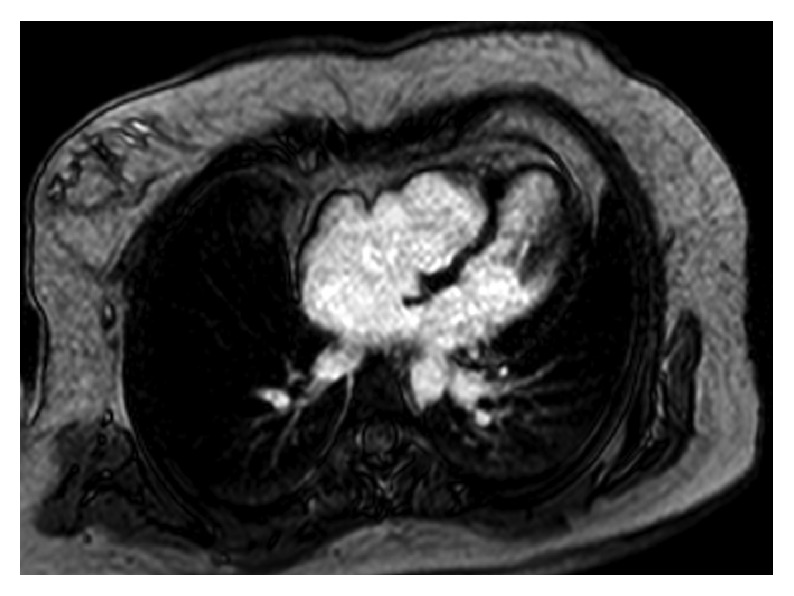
Nonapex-forming RV seen on MRI in twin boy.
